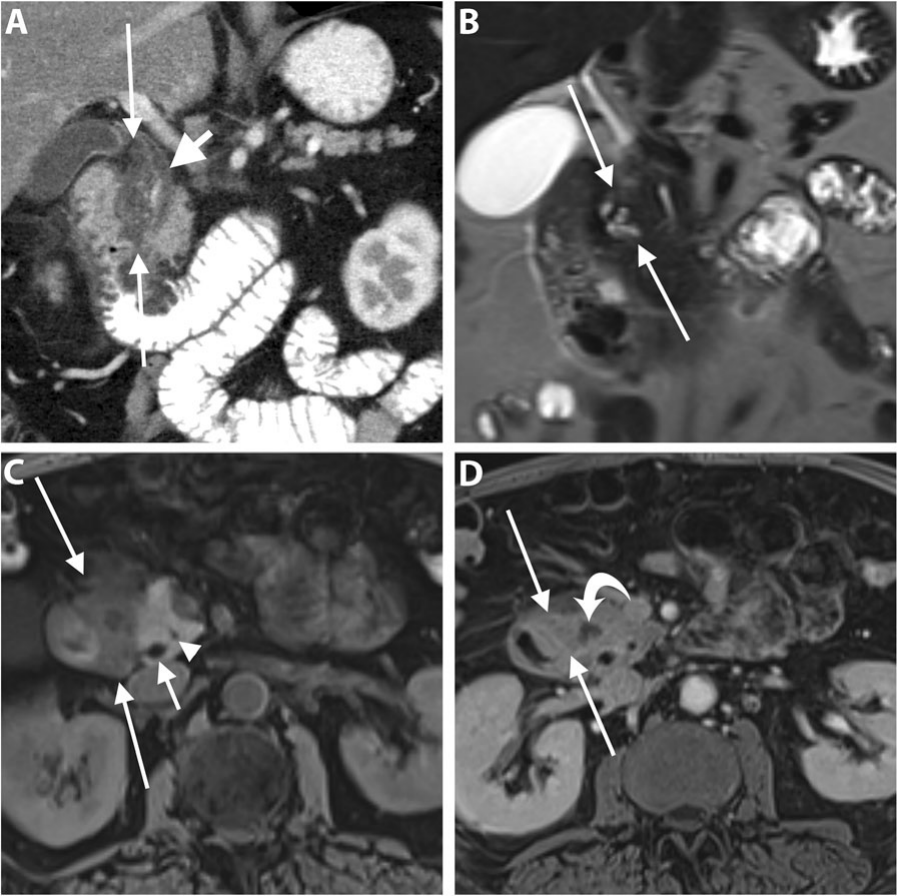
A 60-year-old woman with a segmental form of groove pancreatitis. Coronal portal venous phase CT image (a) shows a hypoattenuating sheet-like area in the pancreaticoduodenal groove (between long arrows) associated with mural thickening and luminal narrowing of the descending duodenum. CBD (short arrow) is displaced medially by the inflammatory process and tapers distally. MR images with coronal T2 HASTE sequence (b), axial fat-suppressed T1 sequence (c), and axial contrast-enhanced fat-suppressed T1 sequence of the delayed phase (d) show the pancreaticoduodenal groove abnormality (between long arrows) containing multiple tiny cysts along the duodenal wall with high T2-signal intensity and a sheet of fibro-inflammatory tissue with low T1-signal intensity, and delayed enhancement. Non-enhancing tiny pseuodocyst is noted (curved arrow). CBD (short arrow) and MPD (arrowhead) are not dilated. The patient has improved on subsequent follow-up
